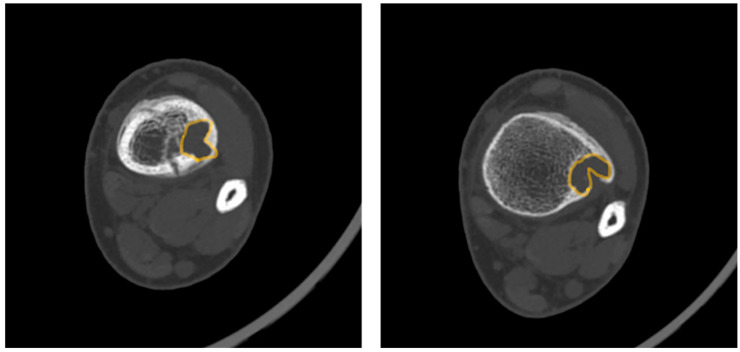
Axial computed tomography slices demonstrating the “Pac-Man Sign” in a 13-year-old boy with a pathologic fracture through a distal tibia NOF. The characteristic shape is highlighted in yellow. The “Pac-Man Sign” is a highly specific (95%) predictor of pathologic fracture, and when present with other high-risk features, prophylactic surgical intervention should be considered.
